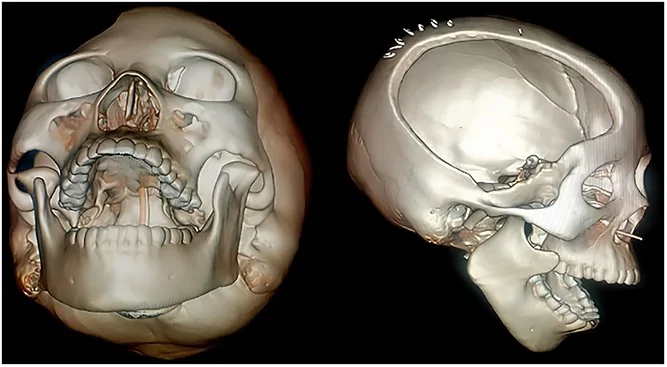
3D CT images showing bilateral anterior temporomandibular joint (TMJ) dislocations in the patient.
